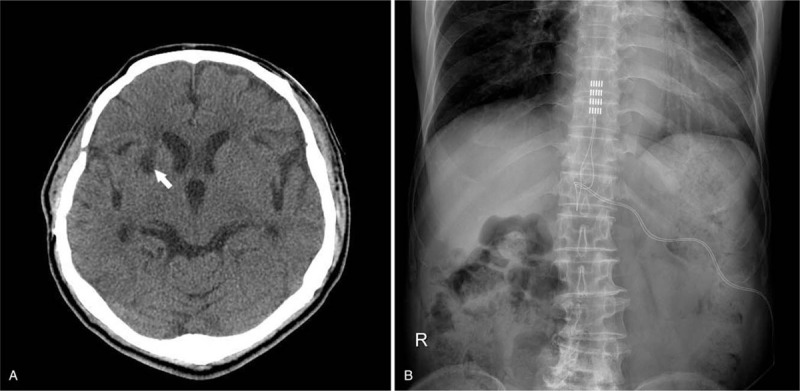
CT and pain film images obtained in Case 2. (A) Axial CT scan shows a right focal hypodense lesion in the basal ganglia (arrow). (B) Plain x-ray shows a paddle electrode placed at the T9 level and an implantable pulse generator.
